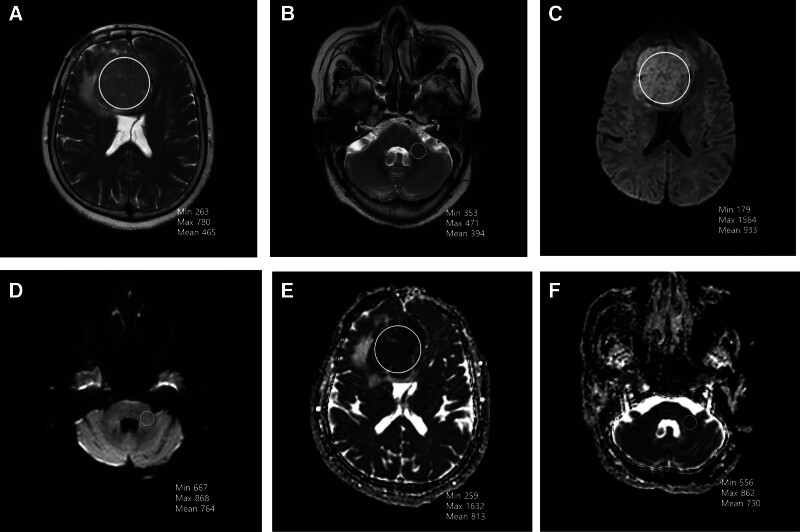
A 78-year-old woman with right frontal falx meningioma (hard meningioma). (A) Tumor ROI on T2-weighted MR sequence. (B) ROI in the middle cerebellar peduncle on T2-weighted MR sequence. The TCTI ratio = intensity value for ROI within tumor/intensity value for ROI within middle cerebellar peduncle = 263/353 = 0.745. (C) Tumor ROI on DWI MR sequence. (D) ROI in the middle cerebellar peduncle on DWI MR sequence. The TCTI ratio = intensity value for ROI within tumor/intensity value for ROI within middle cerebellar peduncle = 179/667 = 0.268. (E) Tumor ROI on ADC MR sequence. (F) ROI in the middle cerebellar peduncle on ADC MR sequence. The TCTI ratio = intensity value for ROI within tumor/intensity value for ROI within middle cerebellar peduncle = 259/556 = 0.465. ADC = apparent diffusion coefficient, DWI = diffusion-weighted imaging, ROI = region of interest, TCTI = tumor-to-cerebellar peduncle T2-weighted imaging intensity.
